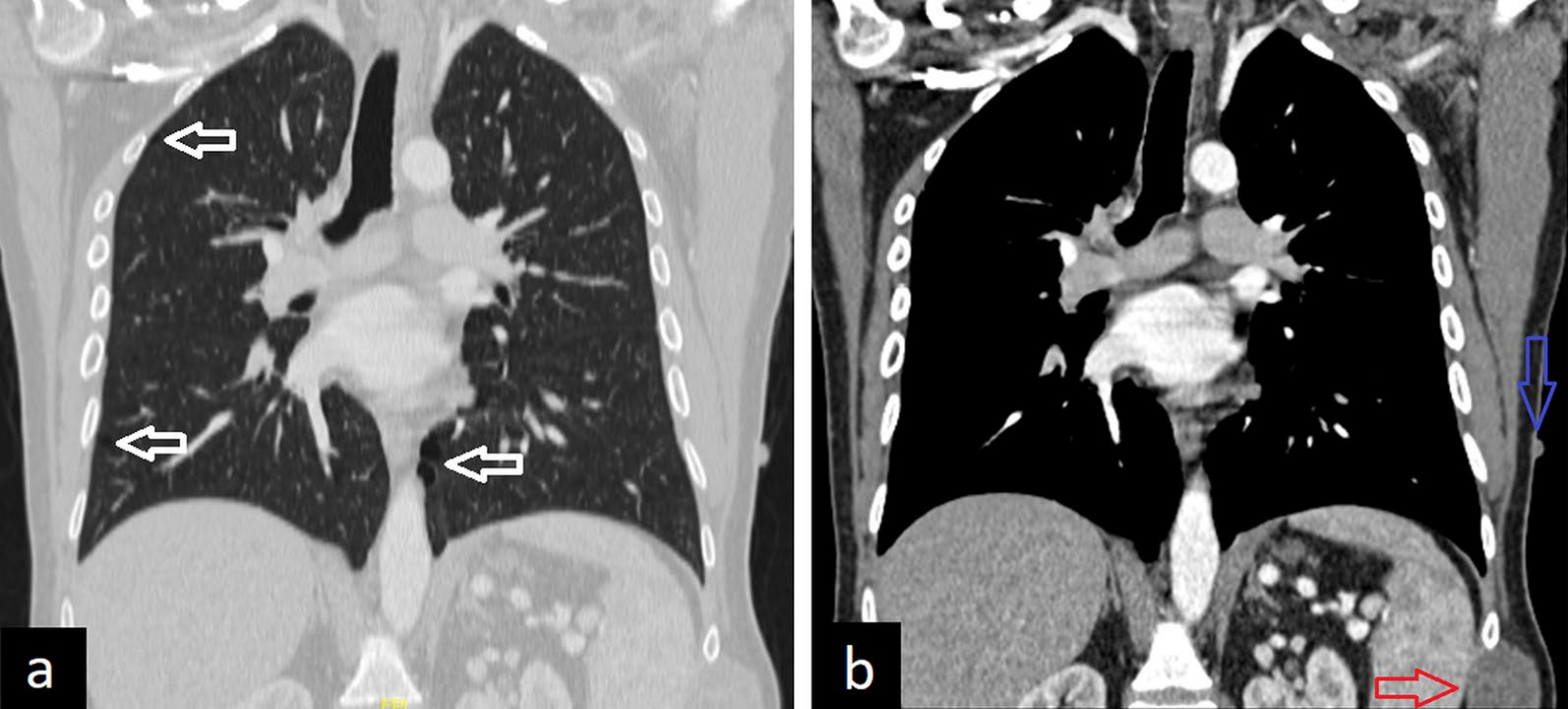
A 32-year-old man with neurofibromatosis type 1. Coronal (a) reconstruction CT image in the lung window shows lung cysts (white arrows). Cutaneous neurofibroma (blue arrow) and neurofibroma arising from the intercostal nerve (red arrow) are seen on the coronal reconstruction image in the mediastinal window (b)
